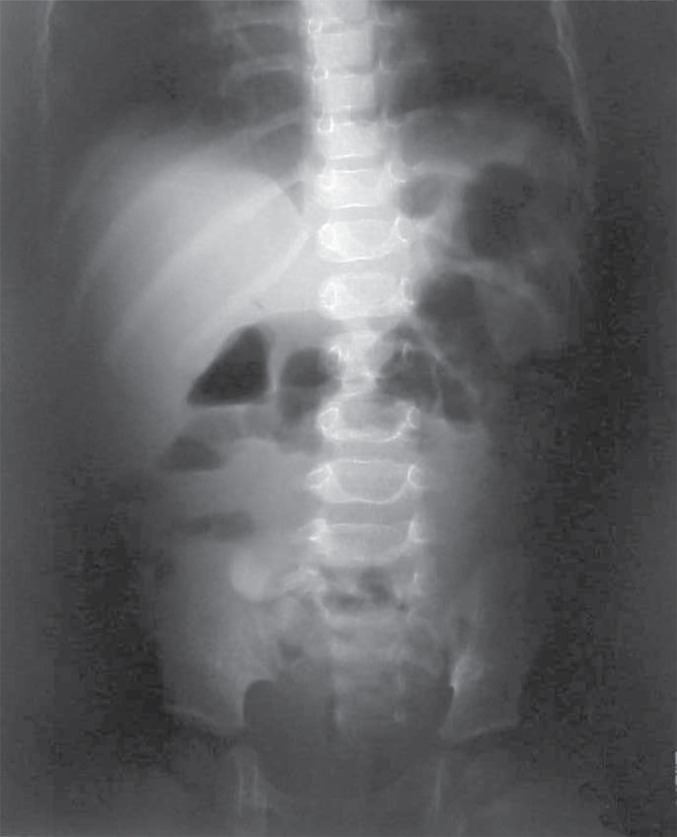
Case No. 1 abdominal X-ray. Imagery was performed two days following hospitalization and revealed a distended left colic flexure.
